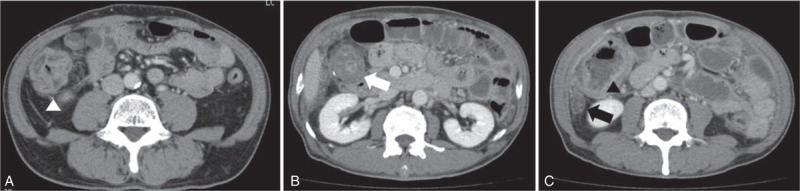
(A) Computed tomography (CT) of abdomen revealed wall thickening of the transverse colon around the hepatic flexure (white arrowhead) at the initial evaluation. (B) and (C) At 20 mo after the initial evaluation, abdominal CT revealed exacerbation of the intestinal stricture in the transverse colon around the hepatic flexure (B, white arrow), dilatation of the ascending colon (C, black arrowhead), and extraintestinal fluid accumulation, which indicated abscess formation (C, black arrow).
